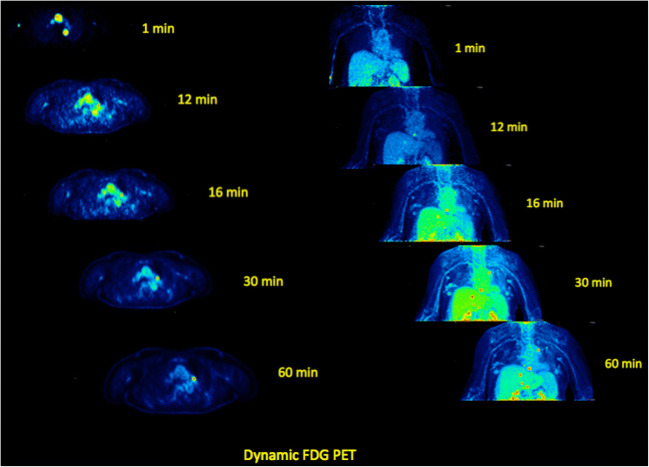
Transversal (left) and coronal (right) images of a dPET series of the thorax following i.v. ^18^F-FDG injection at 1, 12, 16, 30, and 60 min p.i. in a patient with metastases (mediastinal, lung, and liver) from melanoma. Visualization of the vessels in the early images and gradually increasing ^18^F-FDG uptake in the metastases in the following frames
